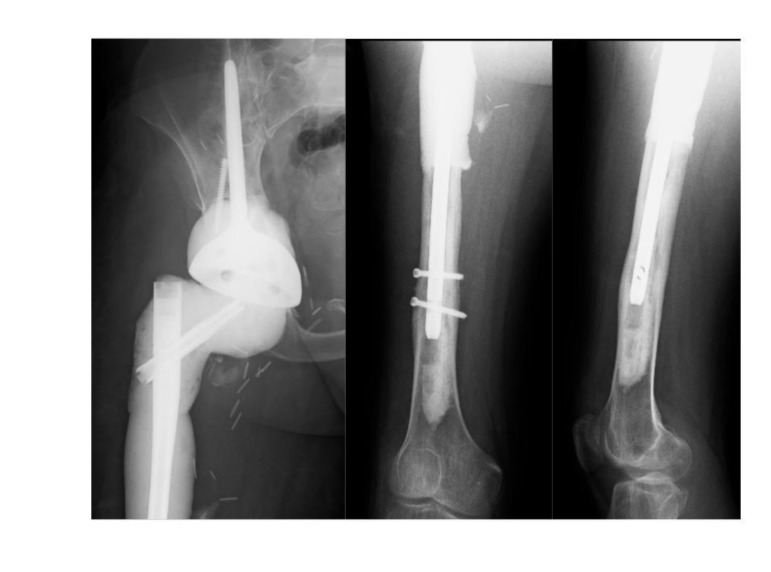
Post operative x-ray of femoral spacer. Note long segment of nail buried into void from previous femoral component stem and preservation of coned pelvis.
